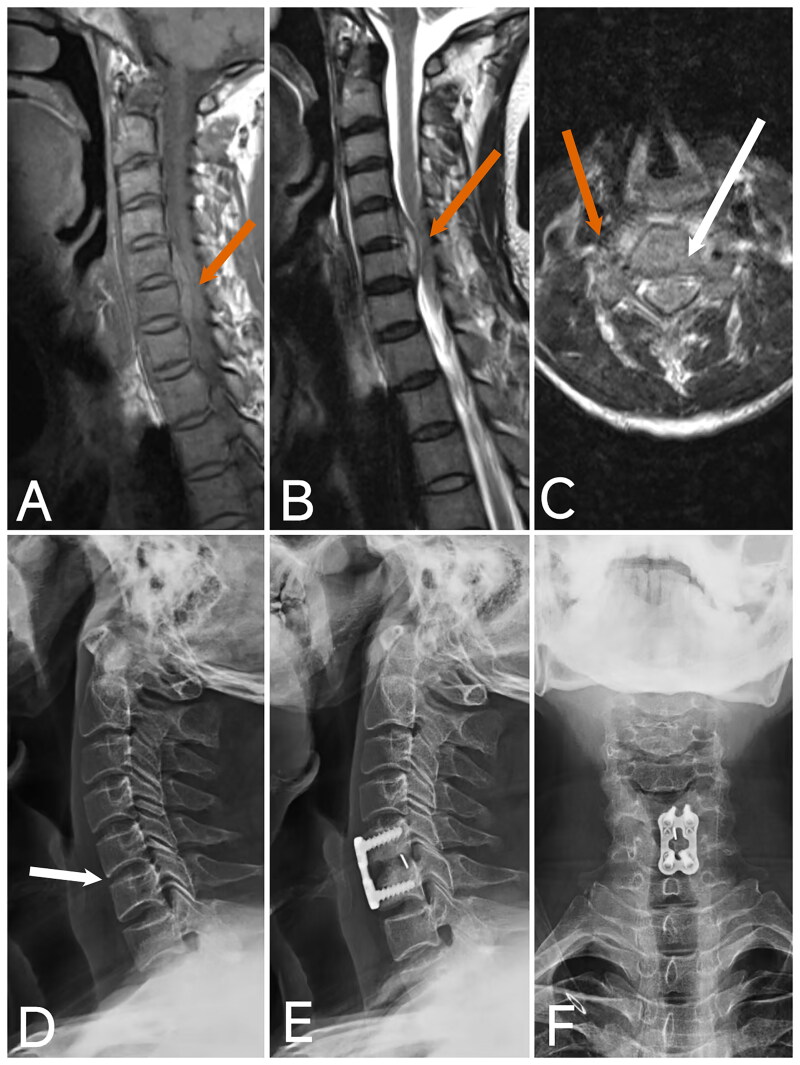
Image of case 4. A: T1-weighted imaging (T1WI); B: T2-weighted imaging (T2WI); C: Axial T2-weighted imaging (T2WI) shows an epidural abscess with significant compression of the spinal cord (red arrow). D: X-ray shows no significant bone destruction or loss of intervertebral height (white arrow). E, F: Postoperative X-ray at 4 months demonstrates good positioning of the internal fixation and fusion of the intervertebral bone graft.
